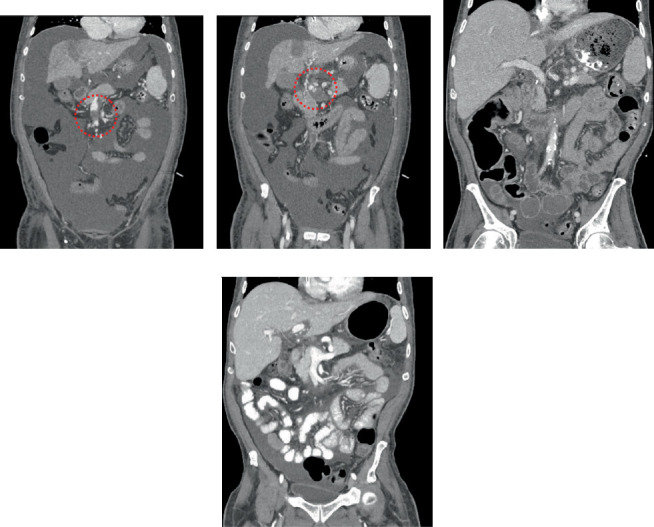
Computed tomography scans pre- and postintervention. (a, b) Scan demonstrating portal vein thrombus extending into the SMV and splenic vein confluence (red dotted circles). (c, d) Three months posttransplant demonstrating trace clot burden and patent splanchnic venous vasculature.
